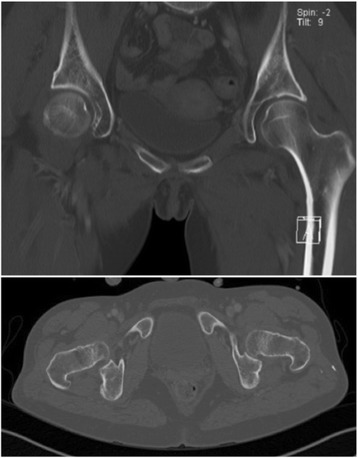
Pre-operative CT coronal and axial views of pelvis and of the proximal femur did not show clear evidence of fracture
